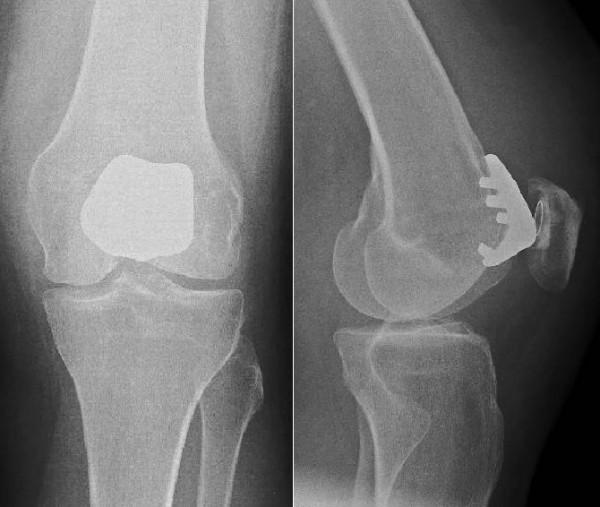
Postoperative radiographs of the left knee with patellofemoral prosthesis in situ.
